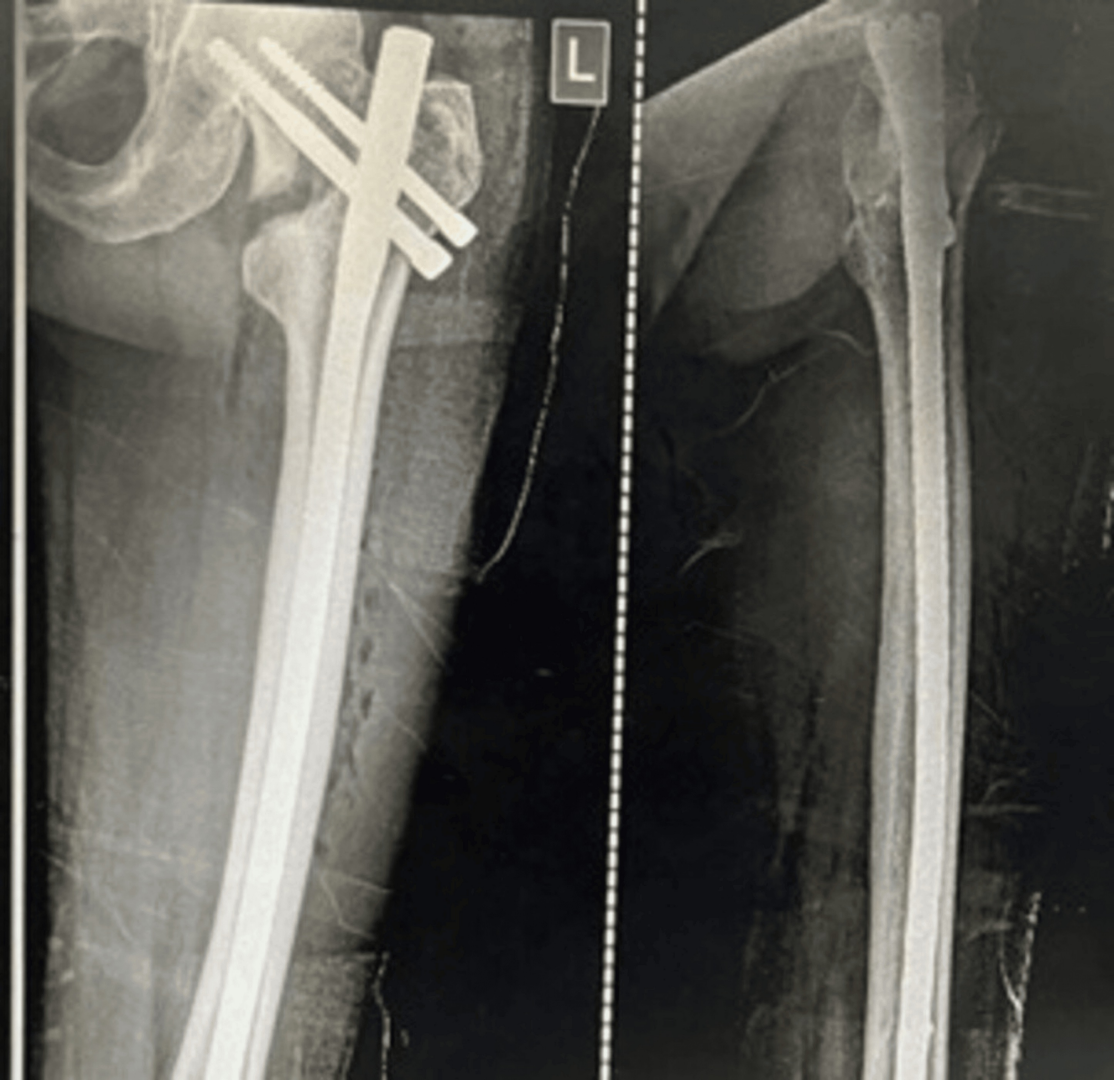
Immediate postoperative AP and lateral radiographs of the same patient treated by a long CMN The patient whose radiograph is shown in Figure 1 was treated with a long CMN. AP: anteroposterior, CMN: cephalomedullary nail.
